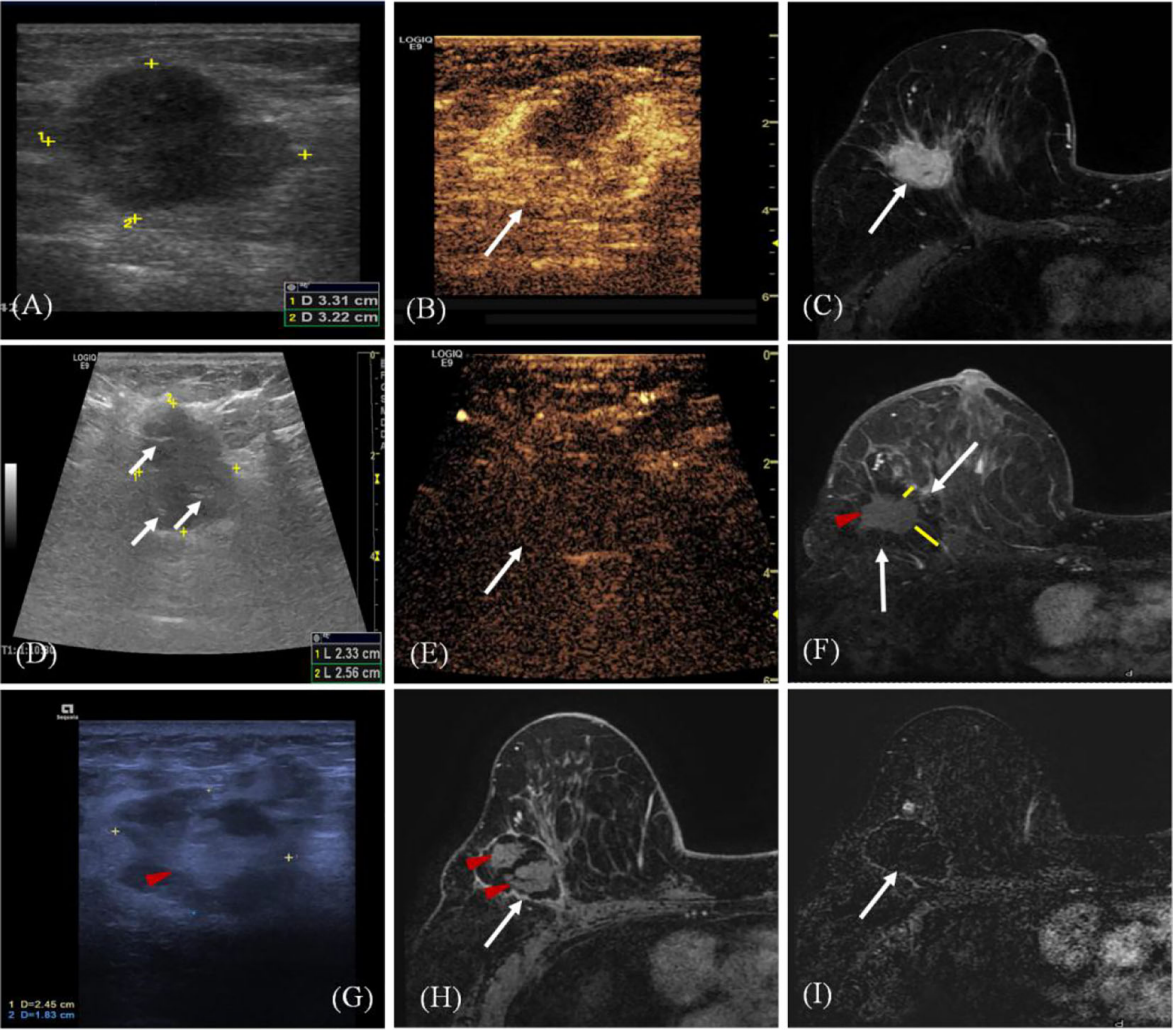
A 68-year old woman with invasive ductal carcinoma of the right breast. (A) Ultrasound (US) scan before microwave ablation (MWA) shows the hypoechoic mass (arrow) with size of 3.3 cm × 3.2 cm. (B) Contrast-enhanced US before MWA shows the mass is hyper-enhanced (arrow) in arterial phase. (C) Transverse contrast-enhanced magnetic resonance imaging (MRI) shows hyperintensity masses (arrow) before MWA in arterial phase. (D) US scan shows the heterogeneously hypoechoic mass (marker) with size of 2.6 cm × 2.3 cm immediately after MWA (ghost size). Hyperechoic needle tracts can be seen in the ablated mass (arrow). (E) Contrast-enhanced US immediately after MWA shows the mass is non-enhanced (arrow) in arterial phase. (F) Contrast-enhanced MRI image shows hyperintensity ghost of mass (red arrow) and the peripheral hypointensity treatment zone (white arrow) in arterial phase three days after MWA. The ablation margin is from 1.2 to 2.2 cm (yellow lines) which can be measured in the hospital information system. (G) US scan shows the heterogeneously ablation zone (marker) shrinks to the size of 2.5 cm × 1.8 cm at 18 months after MWA. Ghost of mass (arrow) is surrounded by hypoechoic adipose tissue. (H) Contrast-enhanced MRI image shows treatment zone (white arrow) is non-enhanced with clear capsule and the central hyperintensity ghost of mass (red arrow) in arterial phase at 18 months after MWA. (I) MRI silhouette shows no signal for the ablation zone with clear fibrous capsule and margin (arrow).
